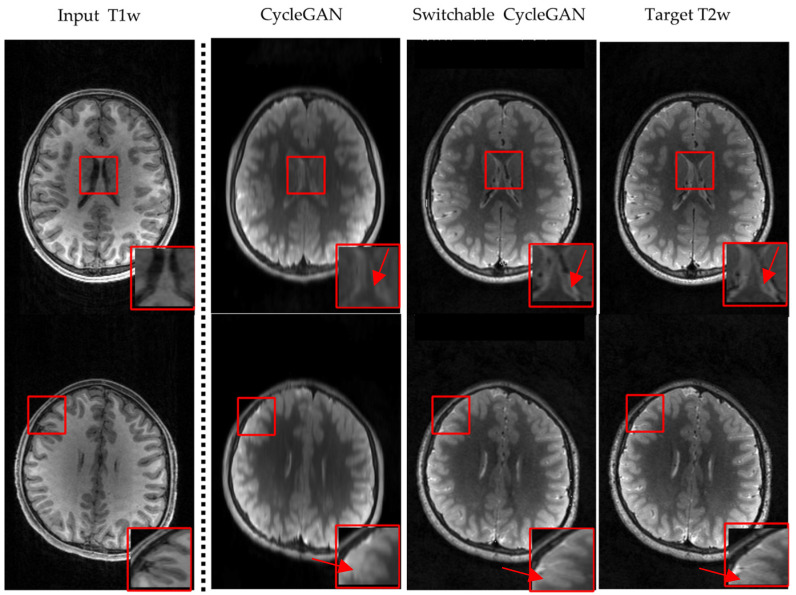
Visual comparison results by CycleGAN and switchable CycleGAN on the ABCD Study Dataset, T1w to T2w image synthesis. Different rows display two individual brain MRI images.
